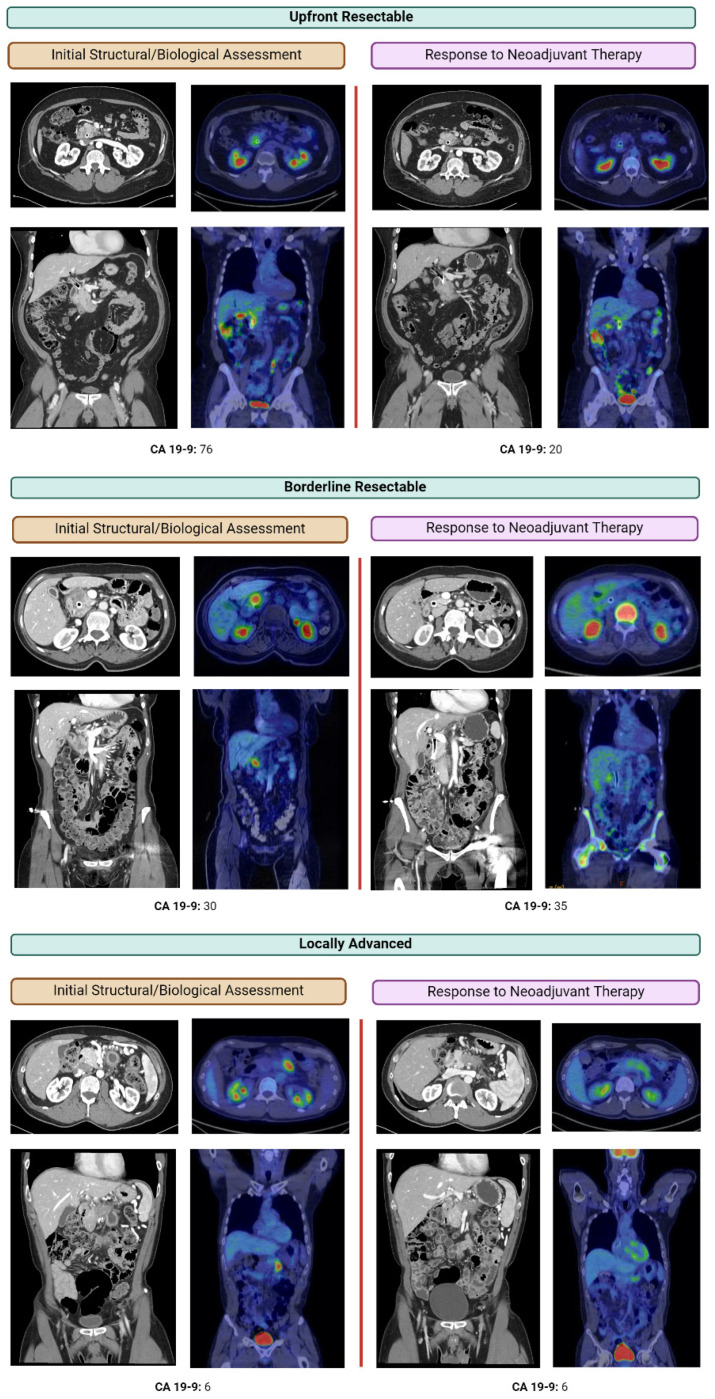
Structural and functional assessment of response to neoadjuvant chemotherapy using CA 19-9 and FDG-PET/CT or FDG-PET/MRI. CA 19-9 and FDG-PET/CT or FDG-PET/MRI before and after neoadjuvant chemotherapy for patients conventionally described as upfront resectable, borderline resectable, or locally advanced disease. Response to treatment is notable as a visual decrease in avidity of the tumor or calculation of SUVmax. FDG-PET scans are particularly helpful in assessment of treatment response of CA 19-9 non-secretors and normo-secretors.
